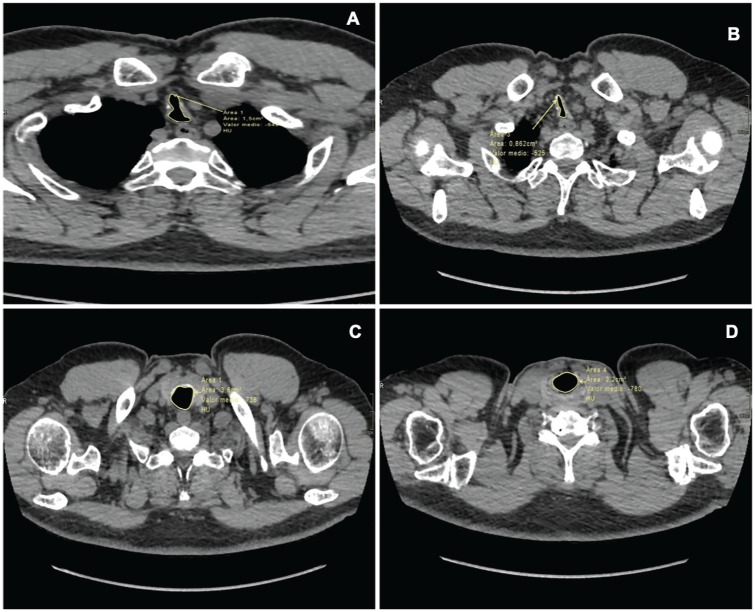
Thoracic axial dynamic computed tomography scan. (A) Demonstrating tracheal stenosis during inspiration with an area of 1.5 cm^2^ that reduces to (B) an area of 0.8 cm^2^ during expiration. (C) Compared with the superior third of the trachea with an area of 3.6 cm^2^ during inspiration and (D) 3.2 cm^2^ during expiration, which gives a stenosis of 75%.
